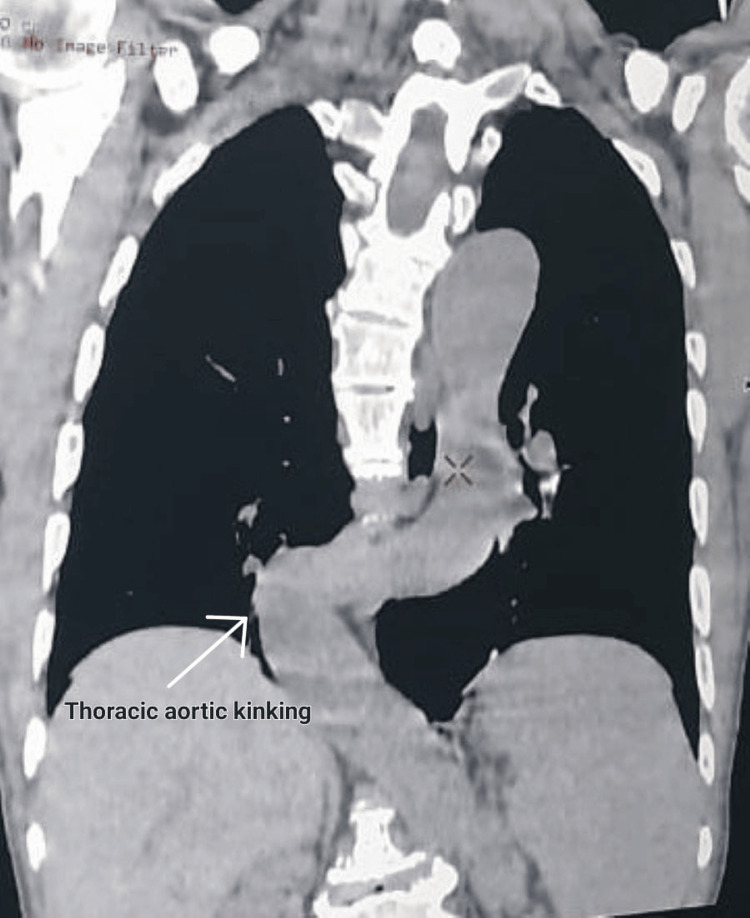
Coronal CT angiography findings The image demonstrates marked angulation (arrow) of the descending thoracic aorta at the level of the aortic isthmus, with preservation of the aortic lumen and no evidence of significant luminal narrowing or collateral circulation. These imaging findings are consistent with thoracic aortic kinking (pseudocoarctation) and help distinguish it from true aortic coarctation CT: computed tomography
